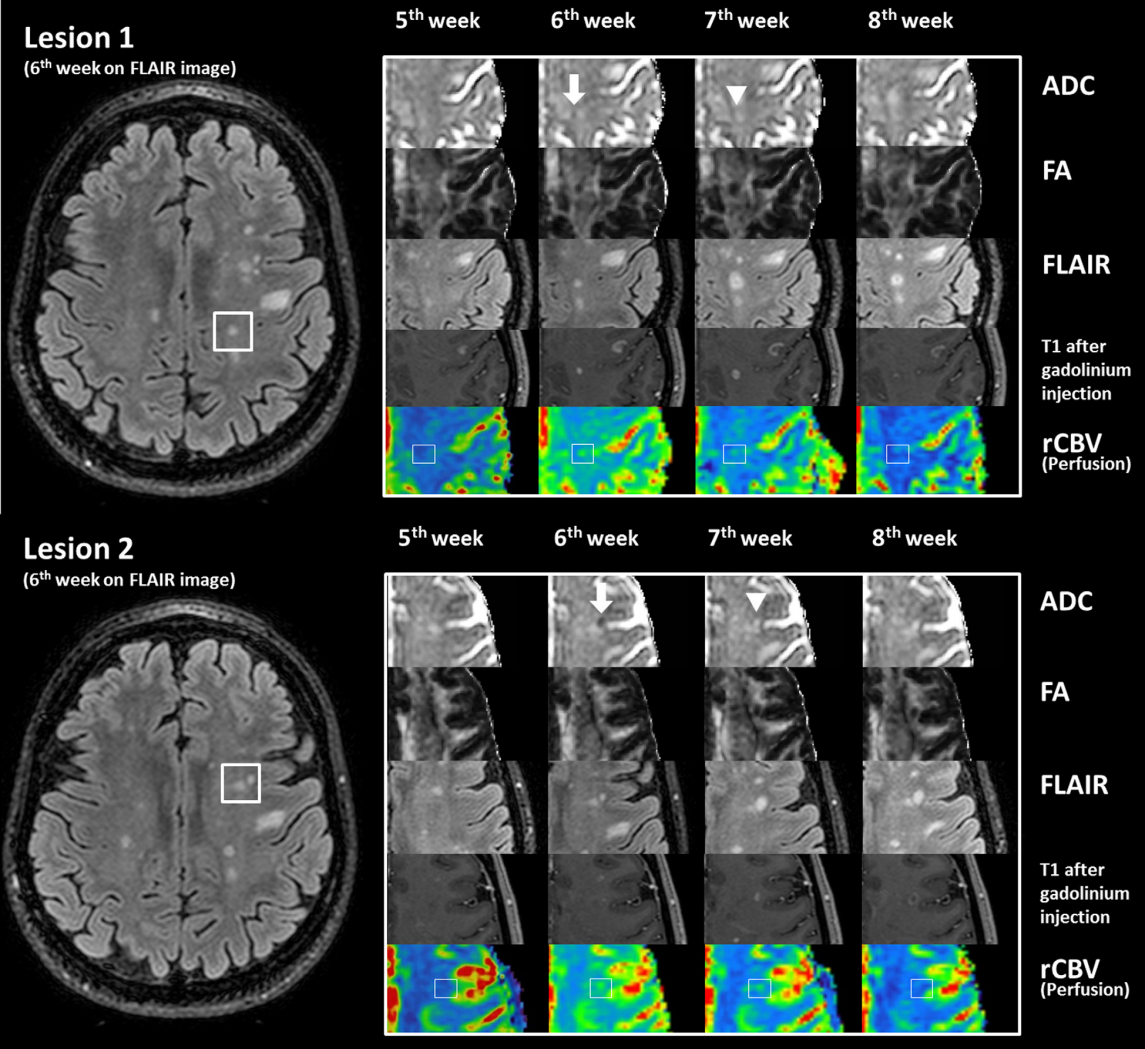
Weekly evolution of both active lesions using multimodal analysis: images of the active lesions at the last four weeks of the examinations seen on ADC and FA maps, FLAIR, T1 after gadolinium injection and rCBV images. Transient decreased ADC and higher rCBV values arised at the 6th week when lesion appeared with injected T1-weighted image (*white arrows*). ADC map of the following weeks shows a marked increase (*white arrow-head*).
